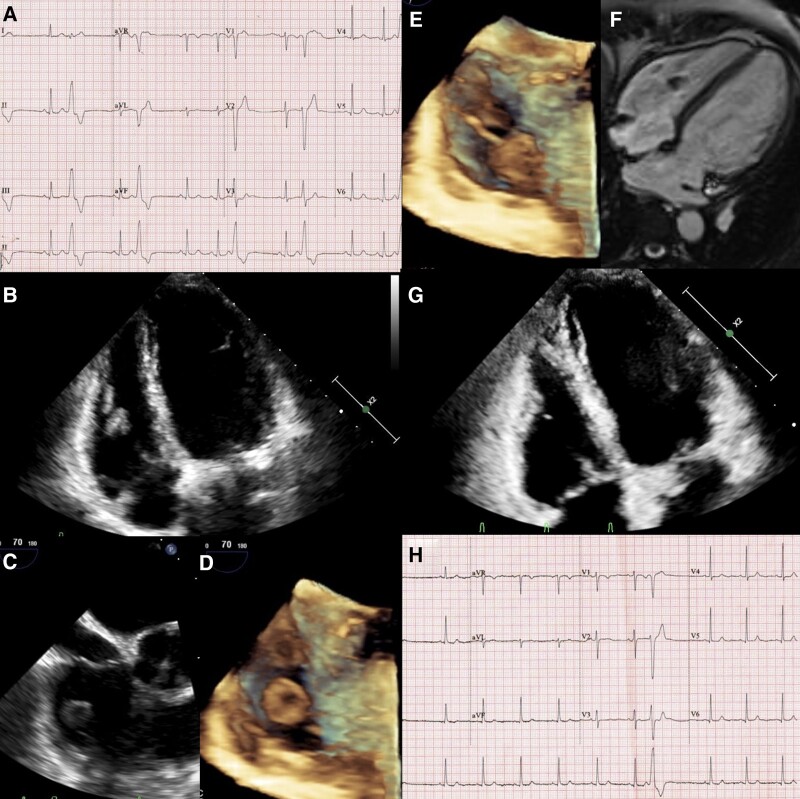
A 44-year-old woman diagnosed with uterine fibroid, admitted for elective vaginal hysterectomy, was referred to us for the evaluation of frequent premature ventricular complexes (PVCs). She had no history of weight loss, diaphoresis, fever, rash, or any cardiac, gastrointestinal, or respiratory symptoms. The PVCs (*Panel A*) were of a left bundle branch abnormality (LBBB) morphology with an inferior QRS axis and a mid-precordial transition—features suggestive of anterior right ventricular outflow tract (RVOT) origin. The 24-h PVC burden was 19%. Transthoracic echocardiography (TTE) showed a mass of 15 × 10 mm, freely oscillating in the right ventricle (*Panel B*). On transoesophageal echocardiography (TEE), the mass was cystic and attached to one of the chordae tendineae with a suspicious stalk (*Panels C–E*; see Supplementary material online, *Videos S1* and *S2*). Biventricular function was normal. On magnetic resonance imaging (MRI), it appeared hypointense, showed no late gadolinium enhancement, and was clearly attached to the papillary muscle via chordae tendineae (*Panel F*; see Supplementary material online, *Video S3*). With a suspicion of hydatid cyst, echinococcal IgG enzyme-linked immunosorbent assay (ELISA) was done and was positive. Contrast-enhanced computed tomography of the thorax and abdomen revealed no additional cysts. Despite the atypical location, the diagnosis of cardiac hydatid cyst was deemed to be highly likely considering the very high specificity of IgG ELISA for the diagnosis of echinococcal infection (98–100%). Considering the patient’s asymptomatic presentation, along with the smaller size and cystic stage of the disease and prior case reports showing successful treatment outcomes using medical therapy alone, the patient was started on extended-release metoprolol succinate (25 mg/day) and albendazole (800 mg/day). After 1 month, her PVC burden had reduced, and TTE showed a significant decrease in the mass size. A second follow-up a month later showed complete resolution of the mass and a decline in PVCs (*Panels G* and *H*) with a 24-h PVC burden of 6%, confirming the diagnosis of cardiac hydatid cyst. The patient completed a total of 12 weeks of therapy to ensure adequate sterilization of the cyst. Follow-up monitoring was continued regularly to detect recurrence.
